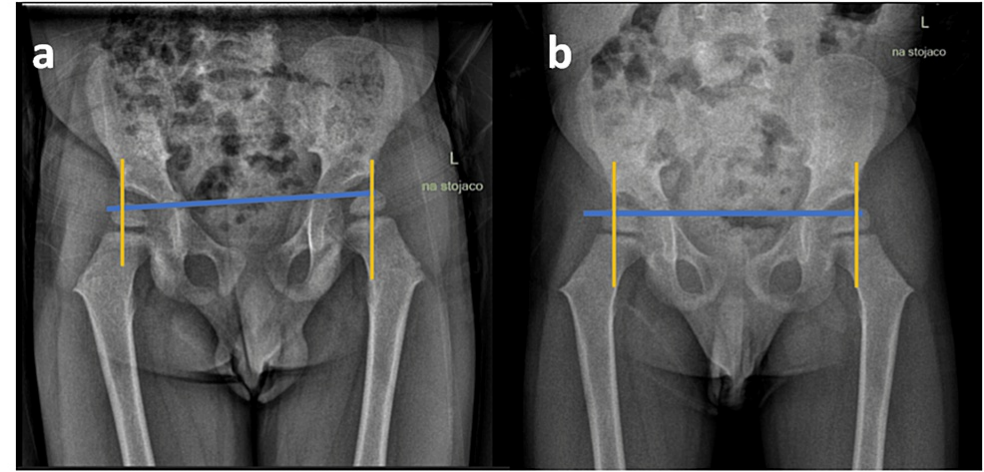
Anterior-posterior (AP) X-ray of the hips pre-treatment (a) and post-treatment (b) Hilgenreiner and Perkin's lines are drawn to evaluate the DDH. The Hilgenreiner line is drawn through the triradiate cartilages. The Perkin's line is drawn perpendicular to the Hilgenreiner line at the margin of the bony acetabulum. The discrepancy in the lower limbs' length is visible DDH: developmental dysplasia of the hip
